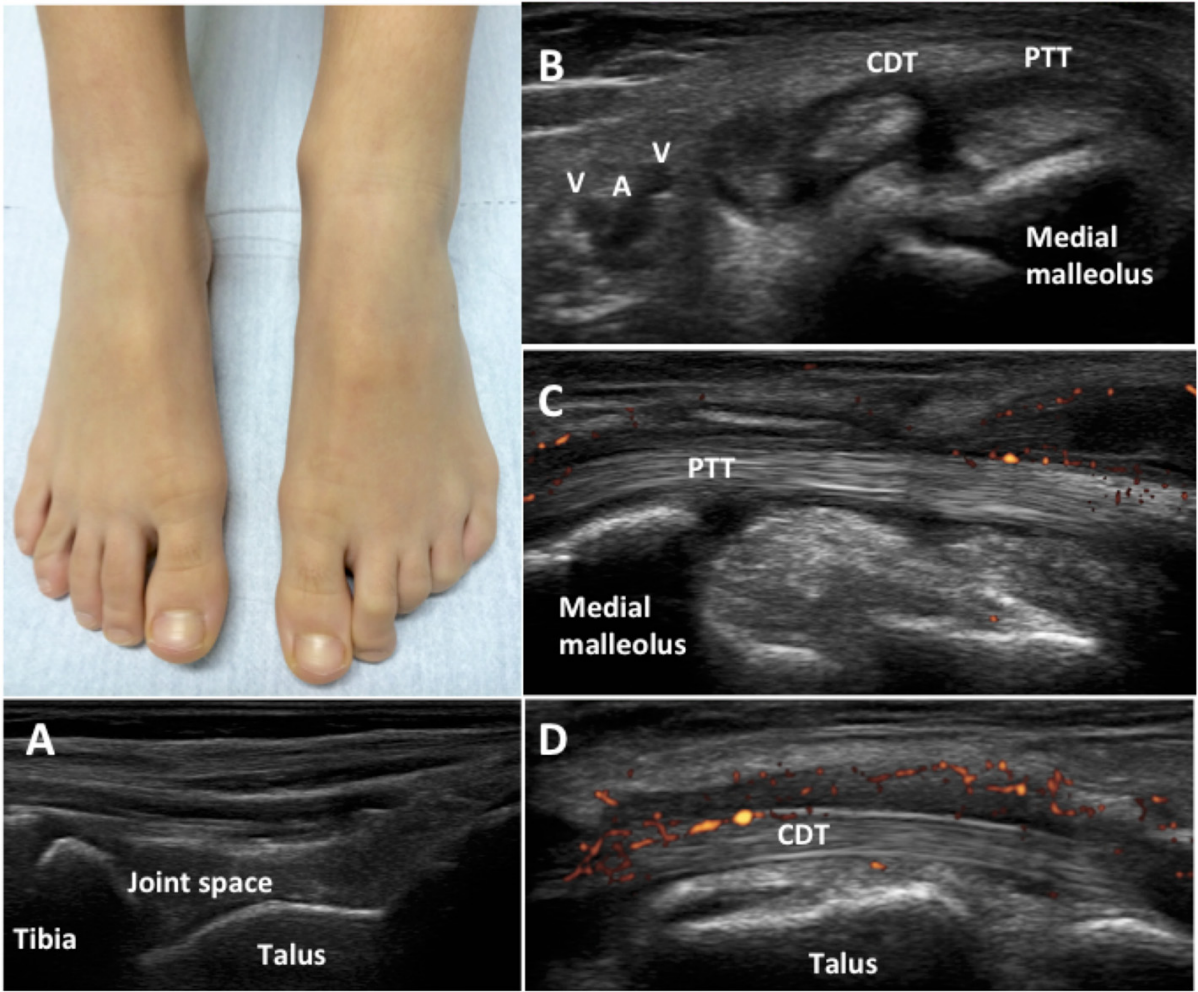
A 10 years old girl with JIA presented with mild swelling of the left ankle, without tenderness/pain on motion or limitation on motion. MSUS showed no signs of synovitis at the tibiotalar joint (a), and allowed detection of a hypo-anechoic halo around the medial tendons (b) and pathologic vascularization on power Doppler along both the posterior tibialis tendon (c) and the common flexor digiti tendon (d), indicating flourishing tenosynovitis. PTT: posterior tibialis tendon; CDT: common flexor digitorum tendon; A: posterior tibialis artery; V: posterior tibialis vein
